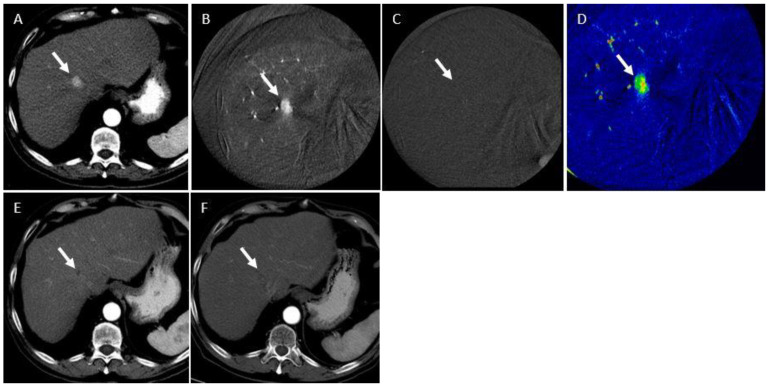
A 79-year-old male with hepatocellular carcinoma (HCC) presents for hepatic artery embolization. (A) Pre-procedural triphasic CT demonstrates one segment 8 lesion (1.8 cm in maximum axial diameter; arrow) with characteristic arterial-phase hyperenhancement, consistent with HCC. (B) Pre-embolization intraprocedural subtracted CBCT demonstrating the hyperenhancing lesion corresponding to the pre-procedural CT (arrows). (C) Post-embolization intraprocedural S-CBCT demonstrating complete absence of tumor enhancement (arrows). (D) ∆ATE map demonstrates a ∆ATE of 45%. (E) One-month follow-up triphasic CT demonstrates complete resolution of hyperenhancement on the arterial phase (arrow). Per mRECIST, treatment response was categorized as complete response (CR). (F) Three-month follow-up triphasic CT demonstrates no local progression or recurrence.
